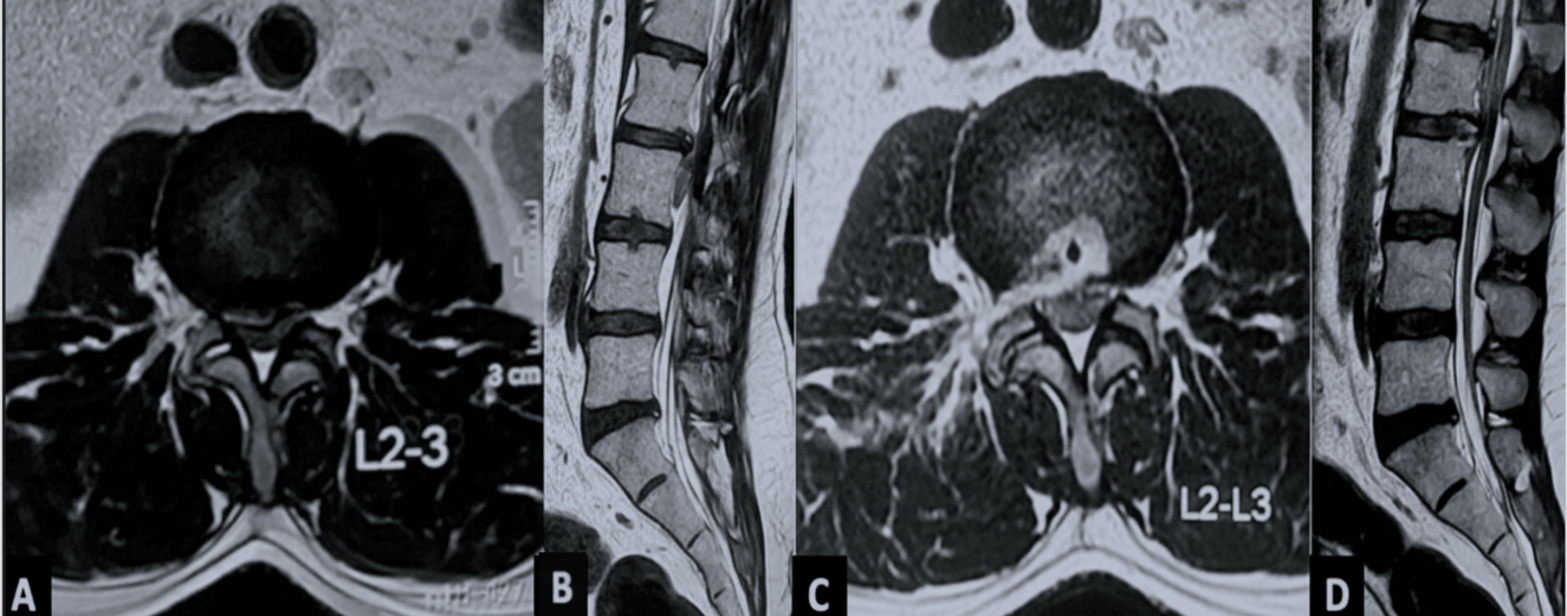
(A, B) Preoperative MRI of a 35-year-old male demonstrating a high-grade downmigrated right-sided disc prolapse reaching the L3 pedicle, classified as Michigan State University type 2AB. (C, D) Postoperative MRI confirming complete fragment removal following the inside-out technique without foraminoplasty.
